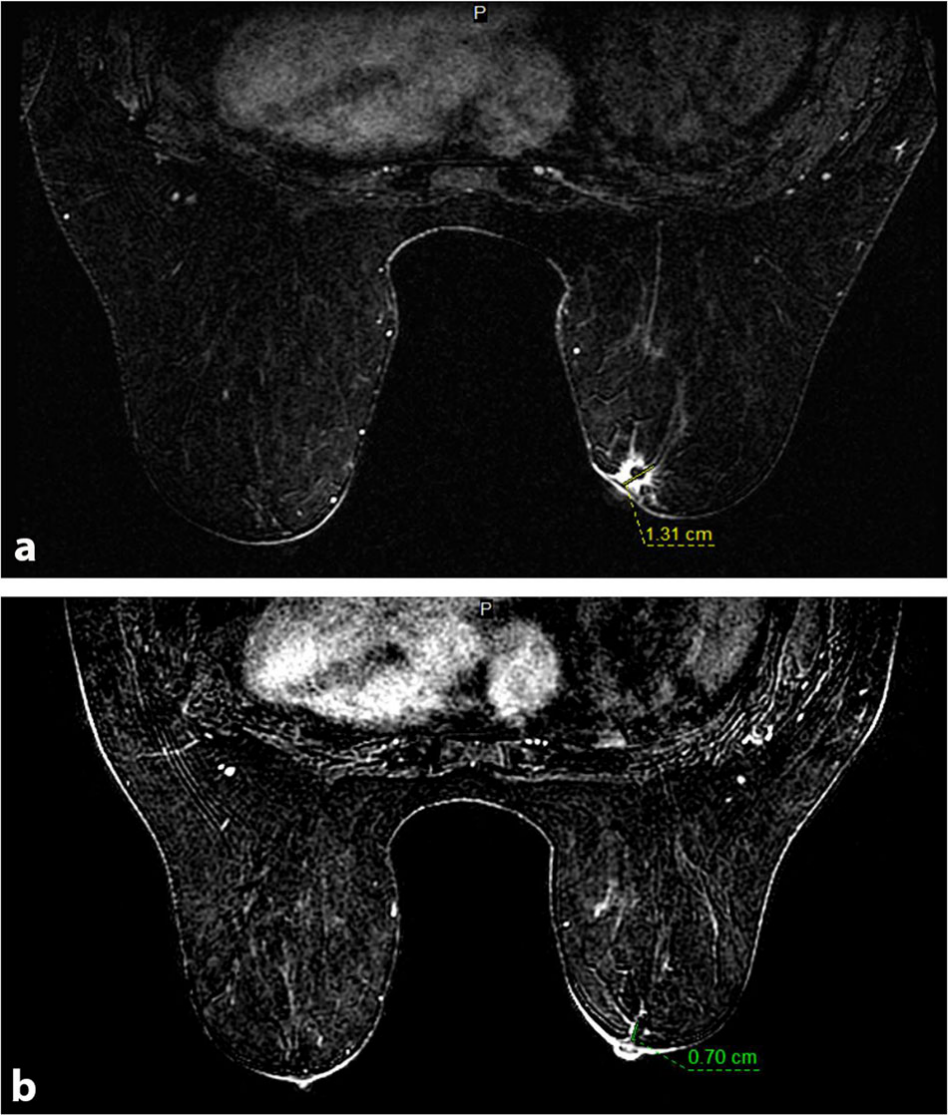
Pre and post neoadjuvant chemotherapy imaging. a Pre-therapy MRI showing retroareolar, irregular enhancing mass, 1.3 cm; b Post-therapy MRI showing faint linear enhancement, 0.7 cm.
